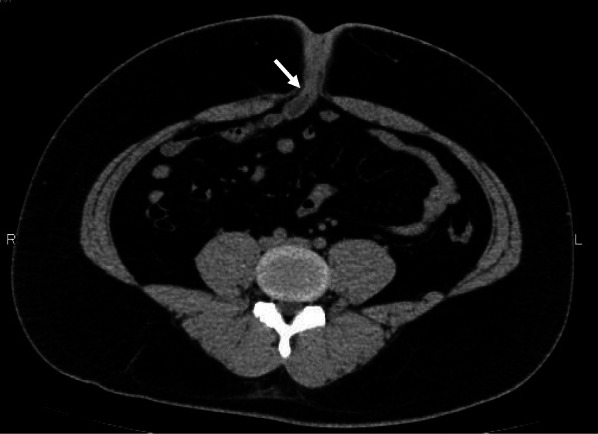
CT image before surgery. Findings resemble those at the first visit, showing a luminal structure with liquid retention just below the umbilicus (arrow)
